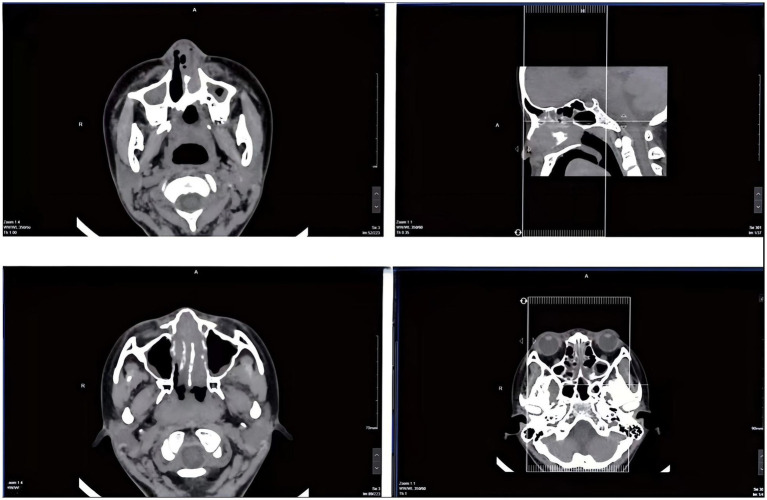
Sinus CT on hospital day 11.
